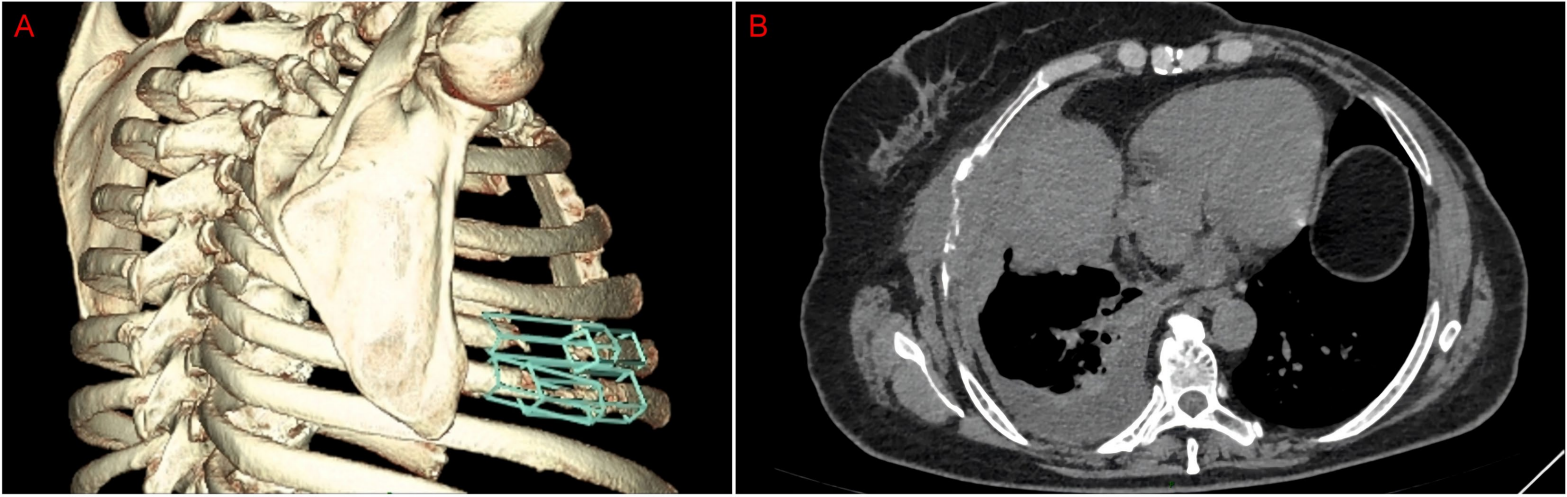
(A) CT images show bone destruction in the right rib. (B) CT shows bone destruction with a soft tissue mass.
